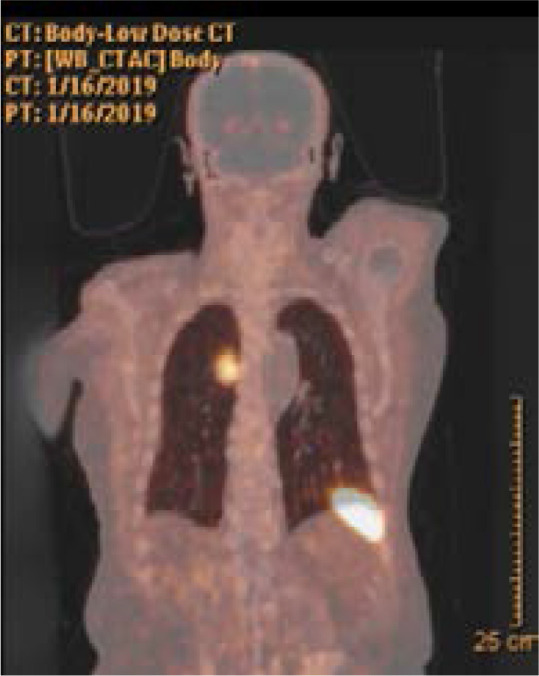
Ga-68 DOTA TATE PET/CT scan image of the lesion, coronal view
